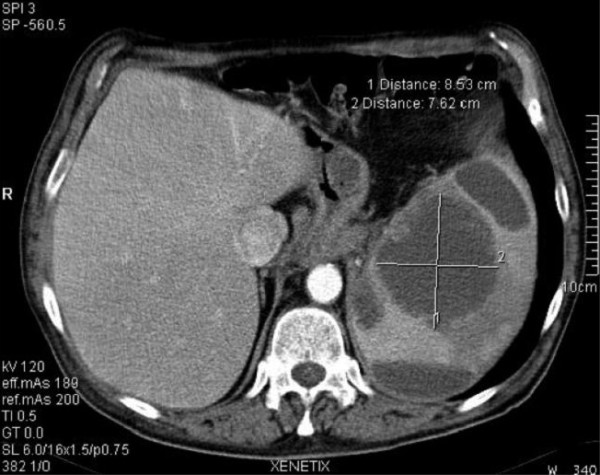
CT-scan showing the largest site of splenic involvement: 8,5 × 7,6 cm.
